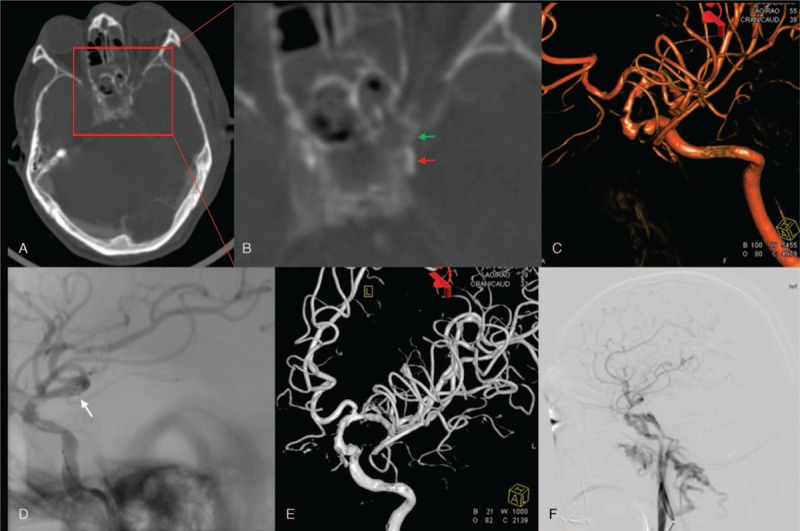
Figures from case 2. (A) The orbit computed tomography (CT) scan and (B) the enlarged figure from red square. The red arrow indicates left internal carotid artery (ICA) and the green arrow indicates fracture flat. (C) The 3-dimensional (3D) reconstructed image of head computed tomography angiography (CTA). (D) The angiography before stent release. The white arrow referes to the distal markers of stent. (E) The 3D reconstructed image after stent release. (F) The DSA follow-up after 3 months, left carotid-cavernous fistula was observed.
